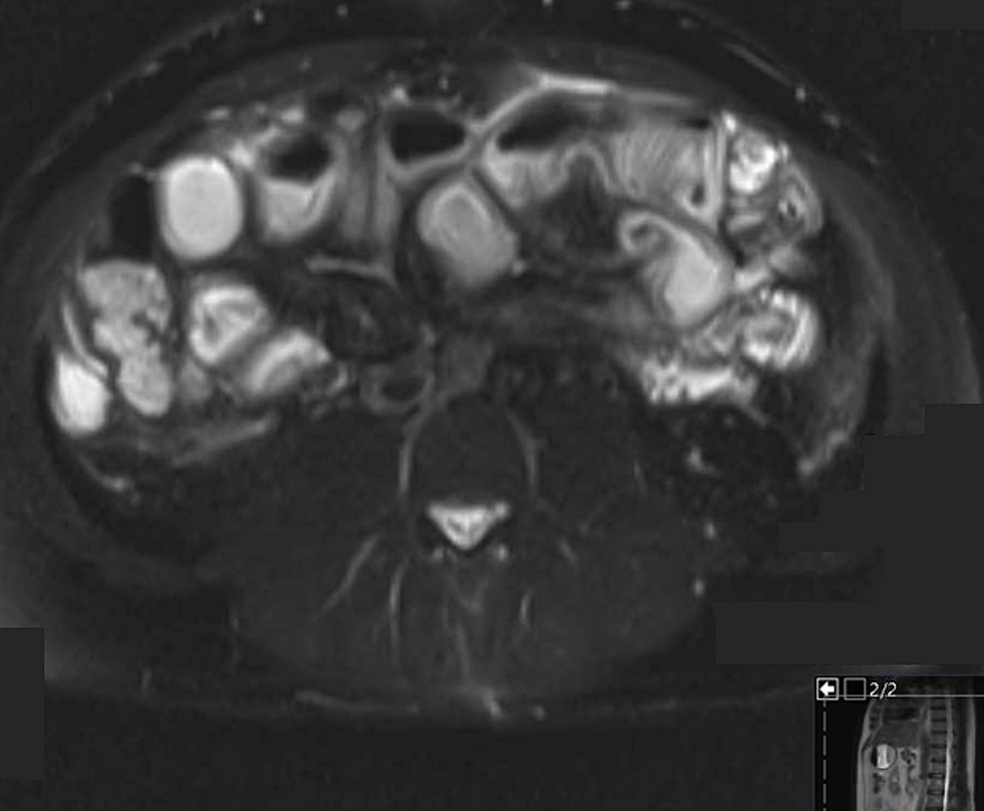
Magnetic Resonance Enterography Magnetic resonance enterography T2-weighted cross-sectional view of the abdomen and pelvis showed multiple loops of the small intestine with wall thickening, edema, and hyperenhancement.
